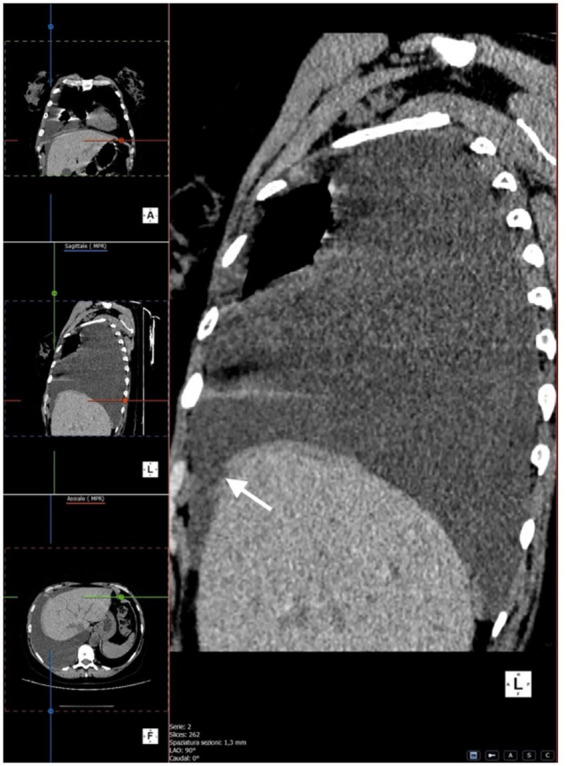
Sagittal CT reconstruction demonstrating a focal area suggestive of diaphragmatic discontinuity of the right hemidiaphragm (white arrow).
